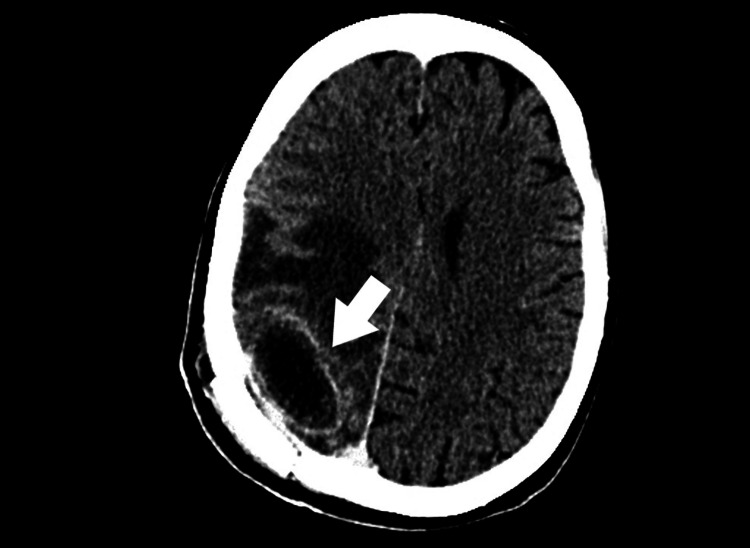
A follow-up CT scan of the head revealed a residual abscess at the surgical site with accompanying edema: a top view (25.02.2017) (marked with a white arrow).
